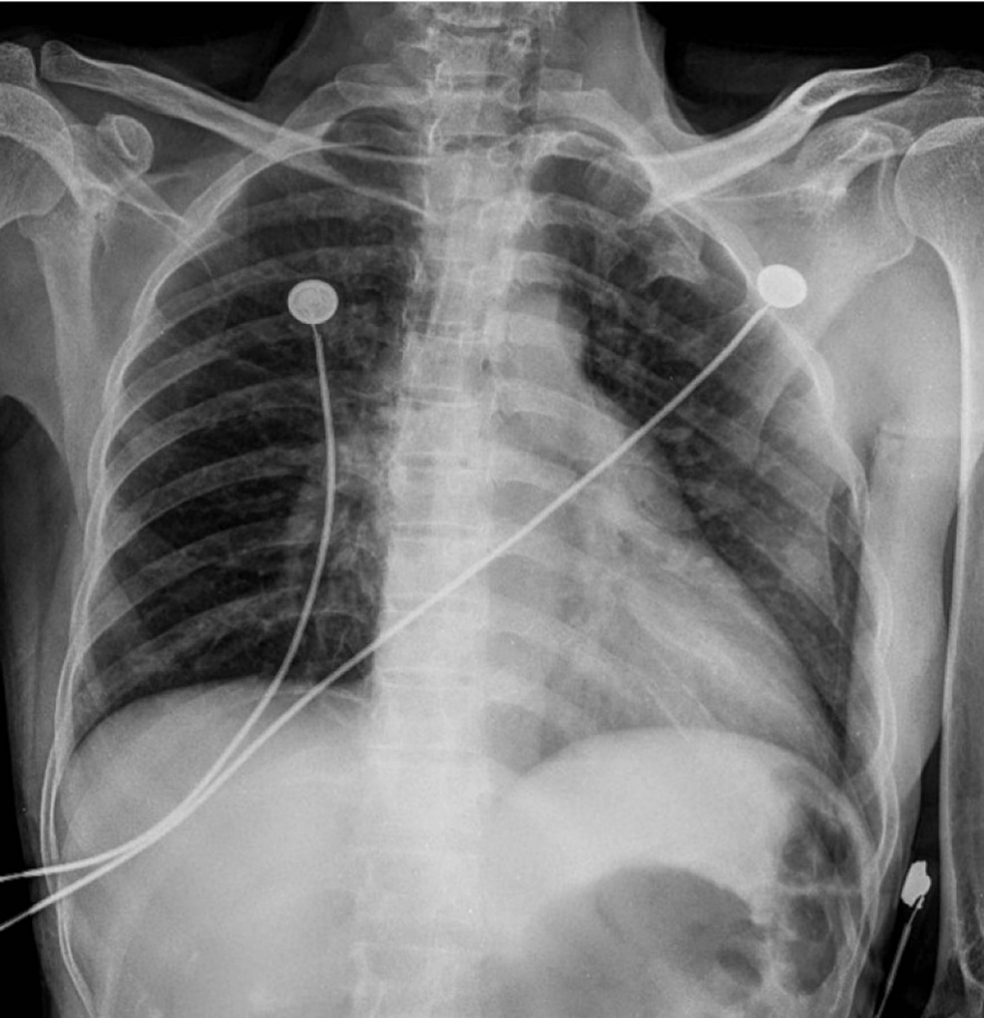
Normal chest X-ray anteroposterior (AP) view. There is no evidence of pulmonary edema.
